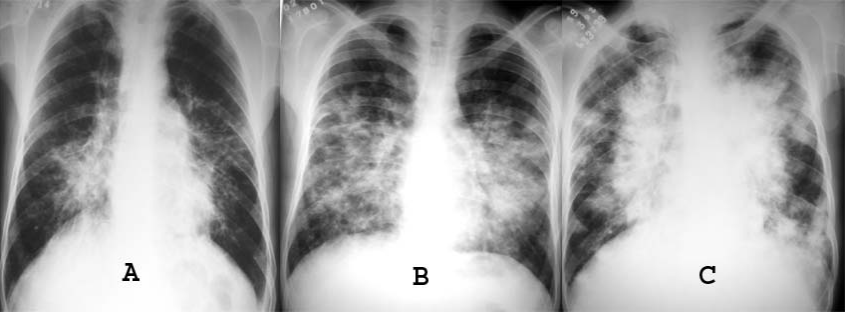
A to C. Chest X-rays of three patients with pulmonary KS showing bilateral paracardiac infiltration. Confluent lesions are most evident in C.
